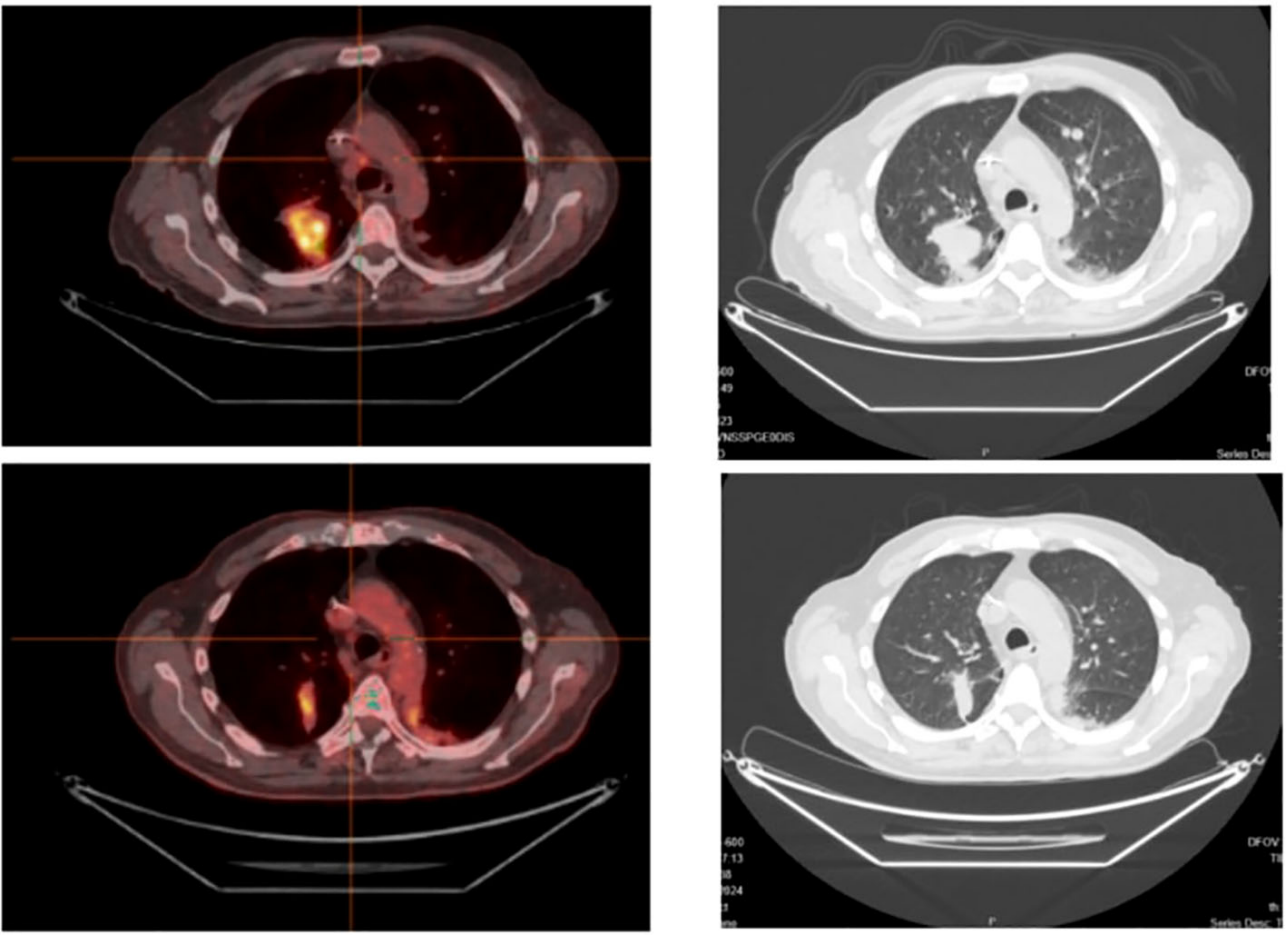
Thoracic response to targeted therapy, on a PET-CT scan (left) and CT-scan (right).
